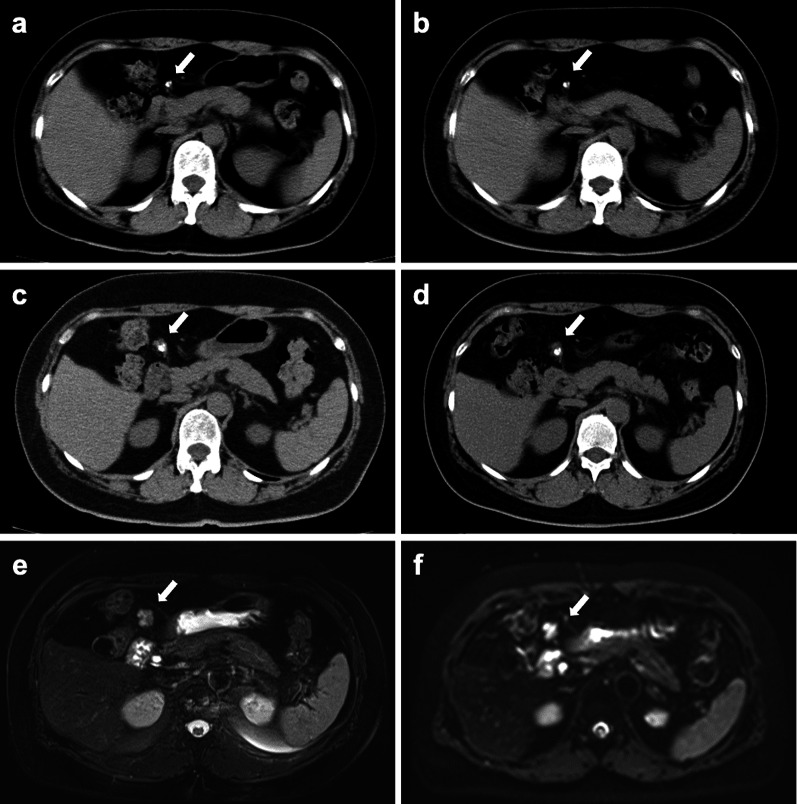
Computed tomography and magnetic resonance images. a–d Computed tomography revealed a gradually growing tumor. e T2-weighted magnetic resonance image showed a faint high-signal-intensity tumor. f Diffusion-weighted magnetic resonance image showed that tumor was high-signal intensity
